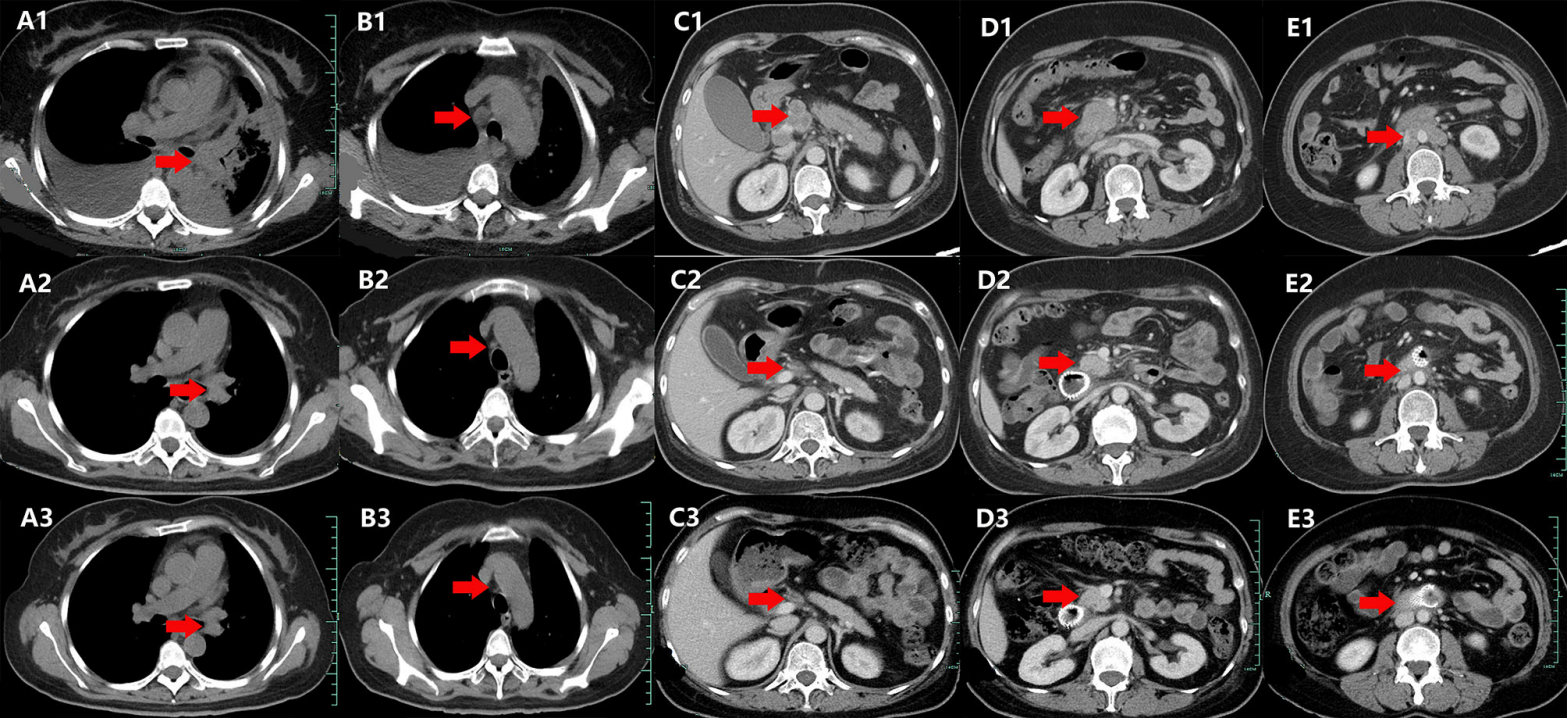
Variations of a space-occupying lesion on retroperitoneum, mediastinum, and left hilar area by CT images during the treatment (red arrowheads). (A1–E1) February 2022. (A2–E2) April 2020. (A3–E3) July 2022.
